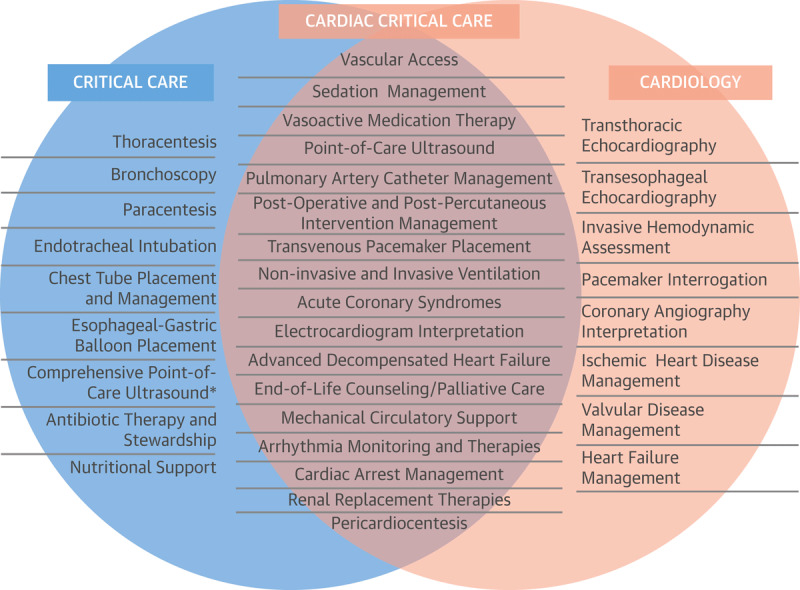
Knowledge and skills for critical care, general cardiology, and cardiac critical care.^9^ Reprinted with permission from Elsevier, Inc. *Lung, abdominal, lower extremity venous ultrasound.
